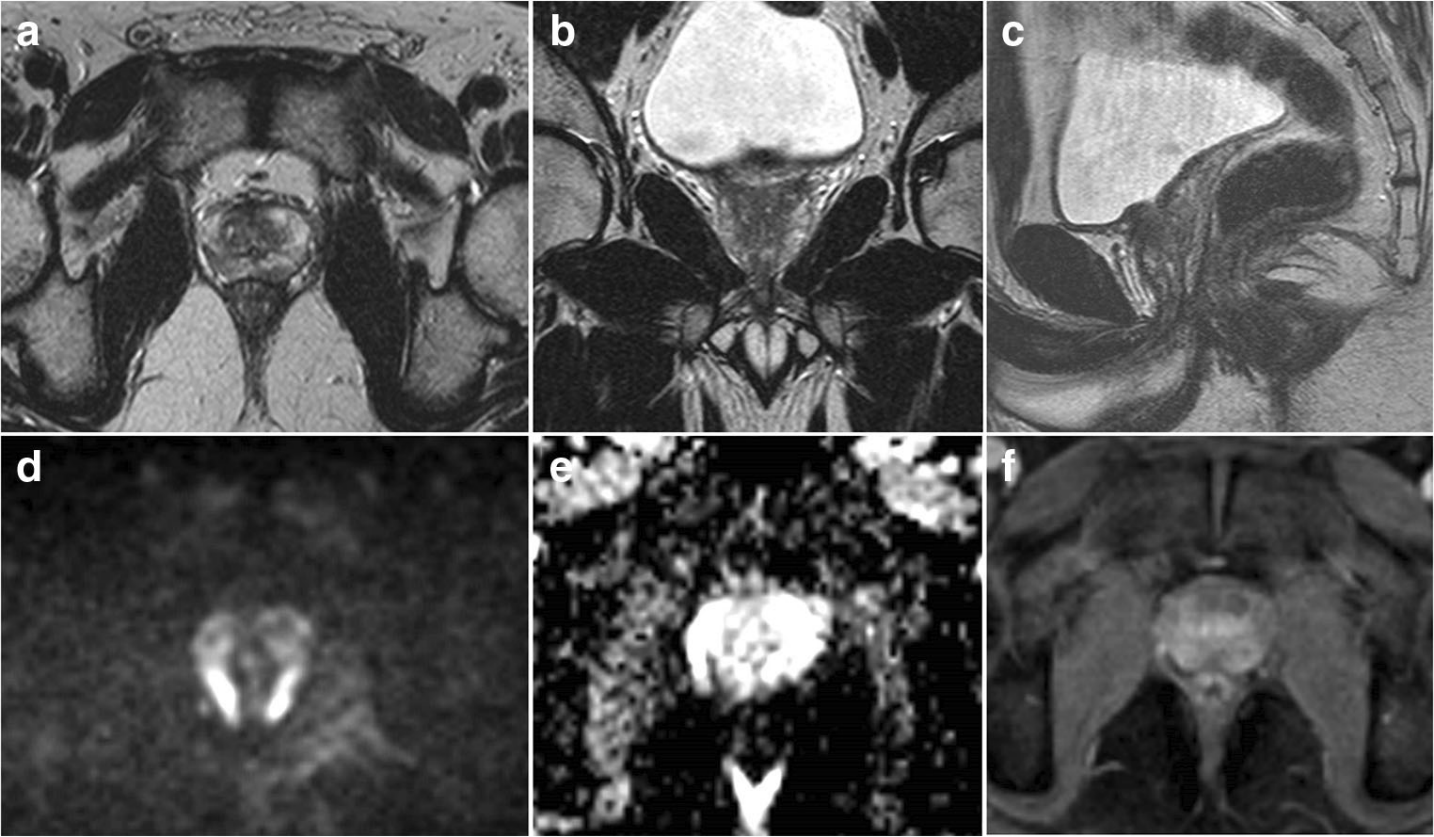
Images of a 50-year-old patient scanned on a 1.5-T MR system with a presenting PSA of 4.7 ng/ml and a prostate volume of 25 cc (PSA density: 0.19 ng/ml/ml), and bilateral Gleason 3 + 4 at biopsy. Axial (a), coronal (b), and sagittal (c) T2-weighted images were judged as the only sequences of acceptable diagnostic quality, as the high *b* sequence (*b* = 1400 s/mm^2^) (d) and apparent diffusion coefficient (e) map from diffusion-weighted imaging showed artefacts from rectal gas and poor in-plane resolution and dynamic contrast-enhanced (DCE) sequences (f) showed suboptimal in-plane resolution and capsular vessels not clearly demarcated. The PI-QUAL score for both readers was 2 (i.e., only one MR sequence is of acceptable diagnostic quality). Final pathology confirmed bilateral Gleason 3 + 4 (pT2c), with an overall tumour volume of 1.7 cc and positive intraprostatic margins on both sides
